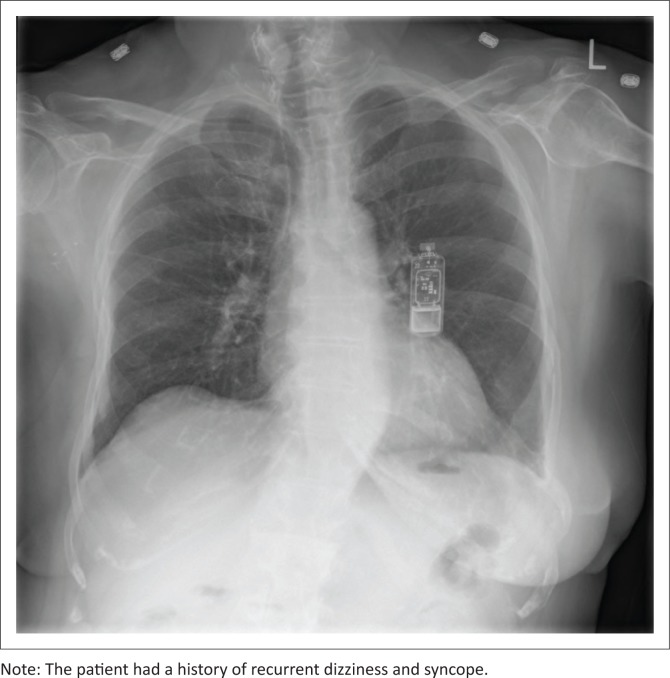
Chest radiograph of a 67-year-old female patient with an implantable loop recorder.
